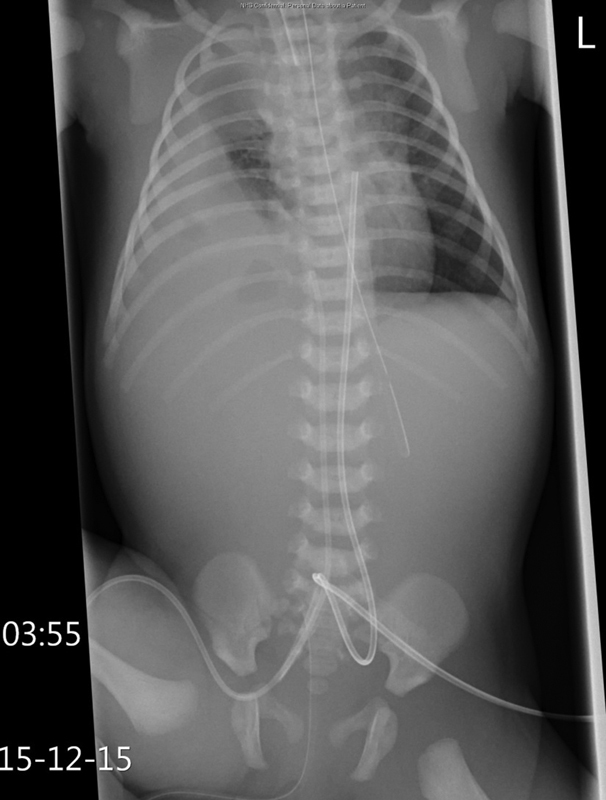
X-ray at birth demonstrating a right-sided CDH. CDH, congenital diaphragmatic hernia.
